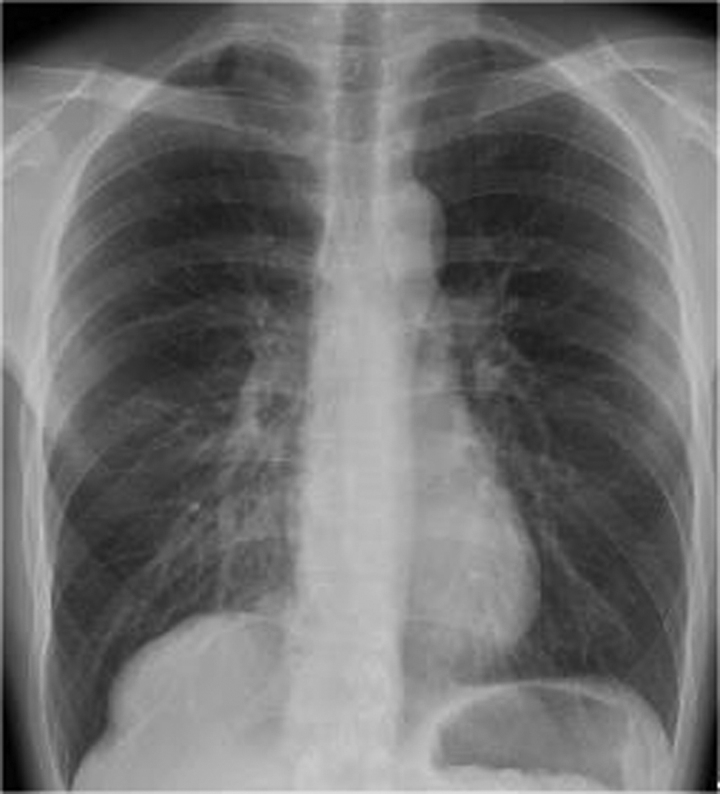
Chest radiograph (frontal view). The right diaphragmatic region shows a tumorous lesion.
